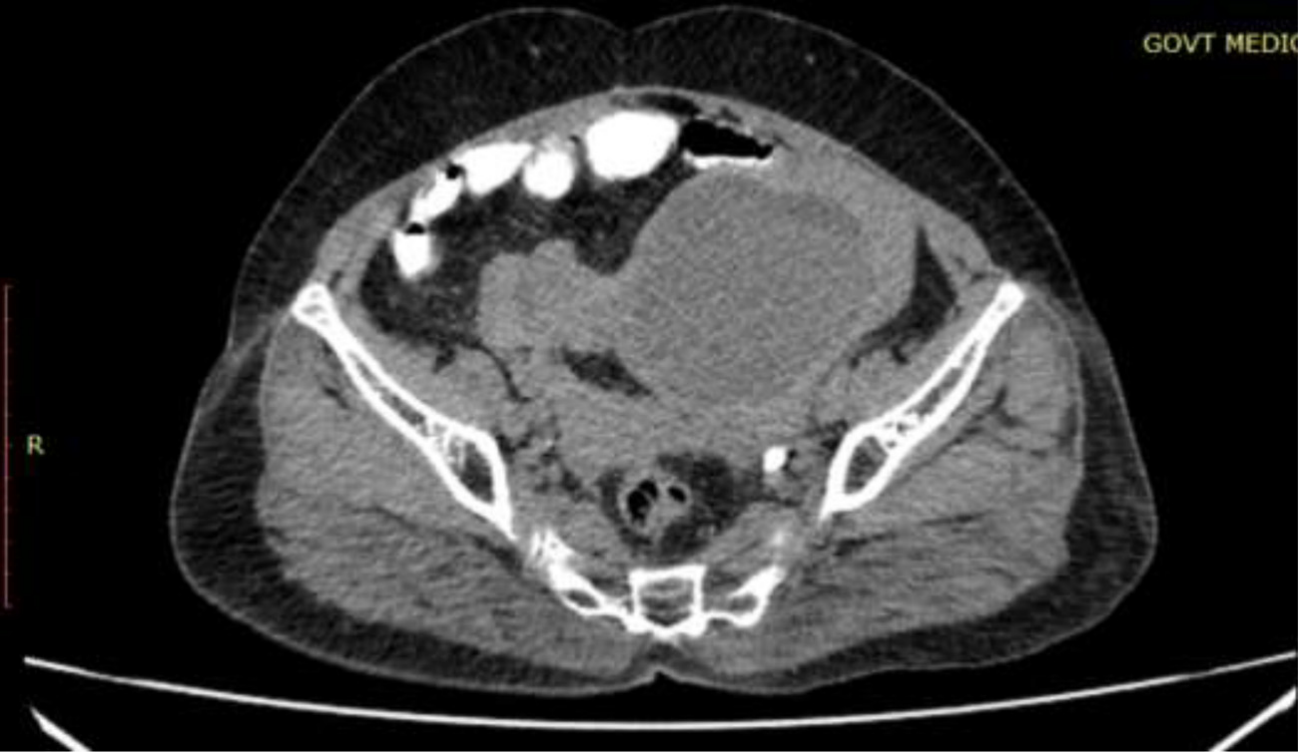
Postcontrast axial CT image shows no significant contrast enhancement of the wall of the lesion as well as the pedicle.
